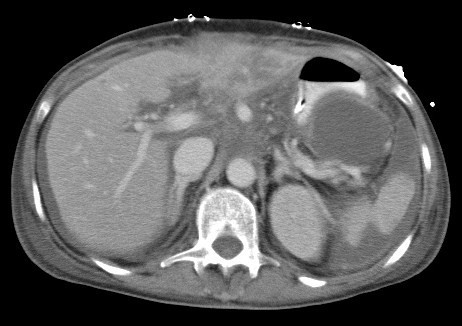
Select axial image of computed tomography showing ischemic necrosis of the left lobe of the liver and infarction of the splenic parenchyma post prosthetic graft occlusion.
